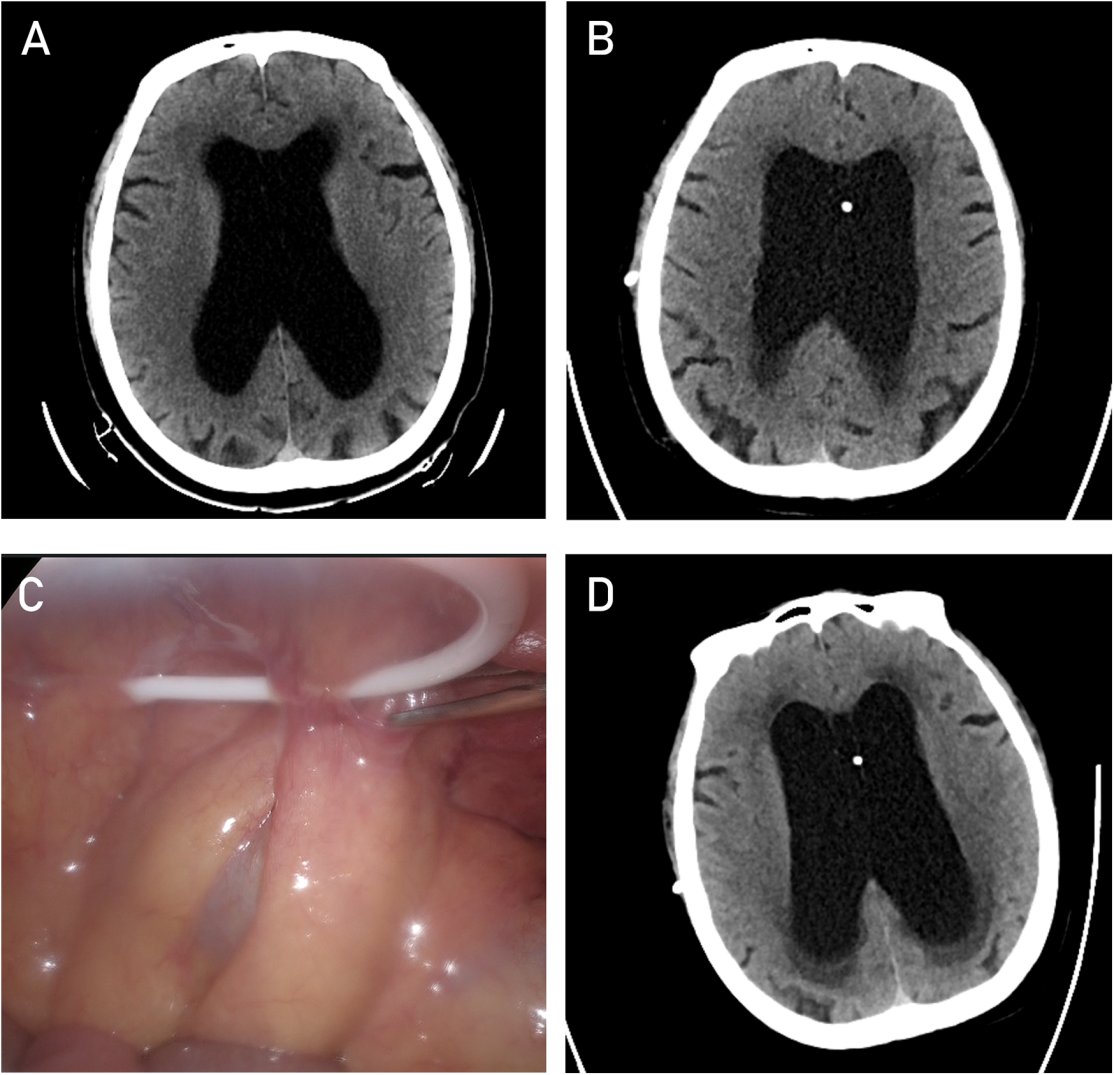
CT image of the lateral ventricles at the time of hydrocephalus diagnosis. (A) CT image of the lateral ventricles after the first VP shunt procedure. (B) Laparoscopic image showing the encapsulation of the peritoneal catheter. (C) CT image of the lateral ventricles after encapsulation of the peritoneal catheter (D).
